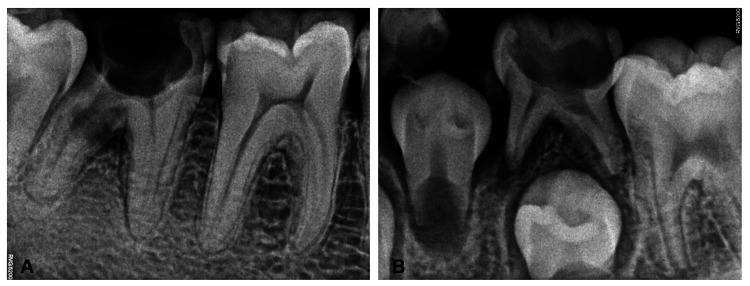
(A and B) Intraoral periapical radiographs taken using a handheld device
